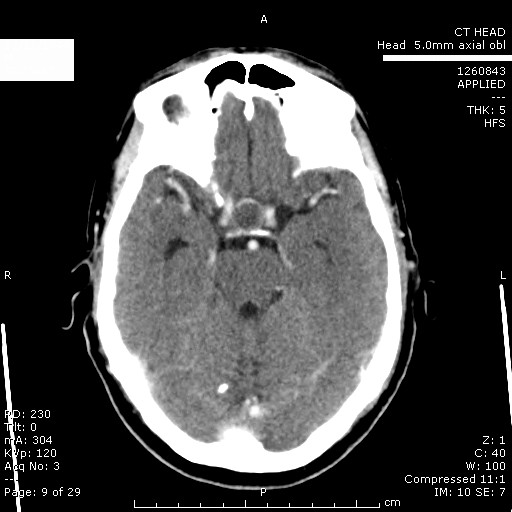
Coronal CT scan showing the pituitary infarct.
